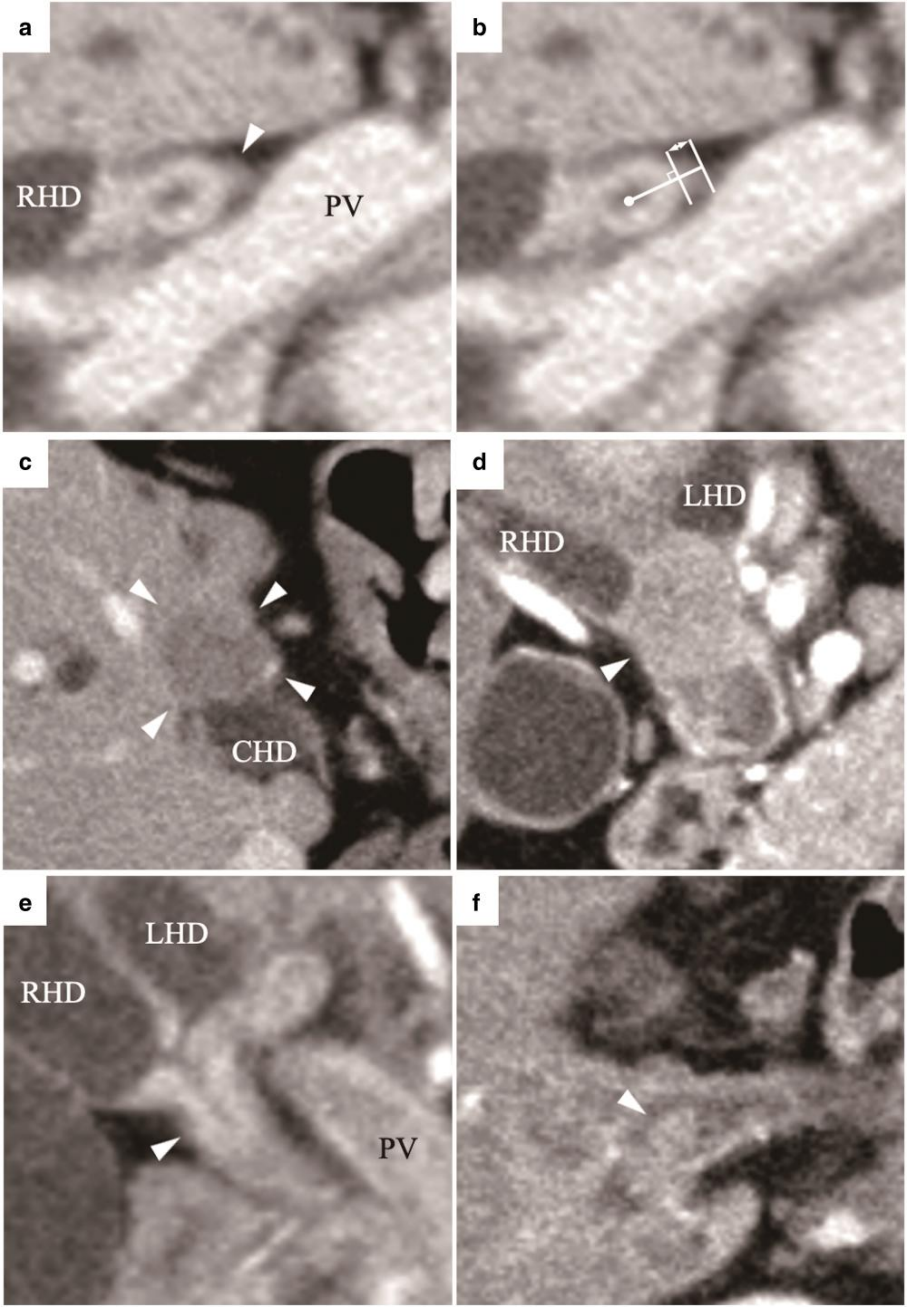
The tumour radiological appearance on multidetector-row CT a The arrowhead indicates the PES. b The length of the PES was defined as the perpendicular distance from the circumference of the bile duct to the vertex of the enation (arrow). c The arrowhead indicates the mass-forming tumour. d The arrowhead indicates a luminal-occlusion tumour. e,f The arrowhead indicates a non-luminal-occlusion tumour. PES, periductal enation sign; PV, portal vein; RHD, right hepatic duct; CHD, common hepatic duct; LHD, left hepatic duct.
